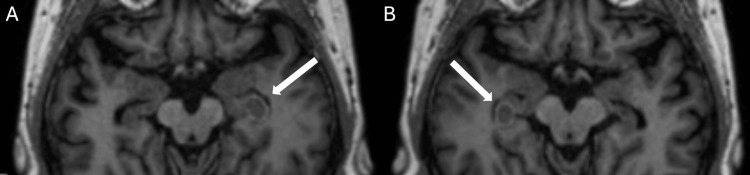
Axial Cranial MRI With Contouring of the Hippocampus in Different Slices A) Left hippocampus and B) Right hippocampus MRI, magnetic resonance imaging
